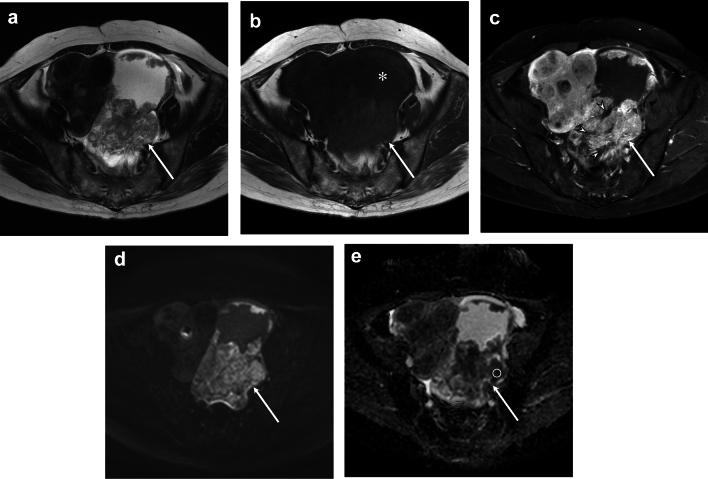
A 52-year-old female with grade 2 OEC. a Axial T2-weighted image showing a cystic mass with heterogeneous solid components (arrow). b Axial T1-weighted image showing a cystic mass with solid components (arrow). The cystic component displays iso-signal intensity relative to the muscle (asterisk). c Axial fat-suppressed contrast-enhanced T1-weighted image showing heterogeneous enhancement of solid component (arrow) with necrosis (arrowhead). d Axial diffusion-weighted image showing a solid component with high signal intensity. e Axial ADC map indicating restricted diffusion (ADC value; 0.97 × 10^−3^ mm.^2^/sec)
